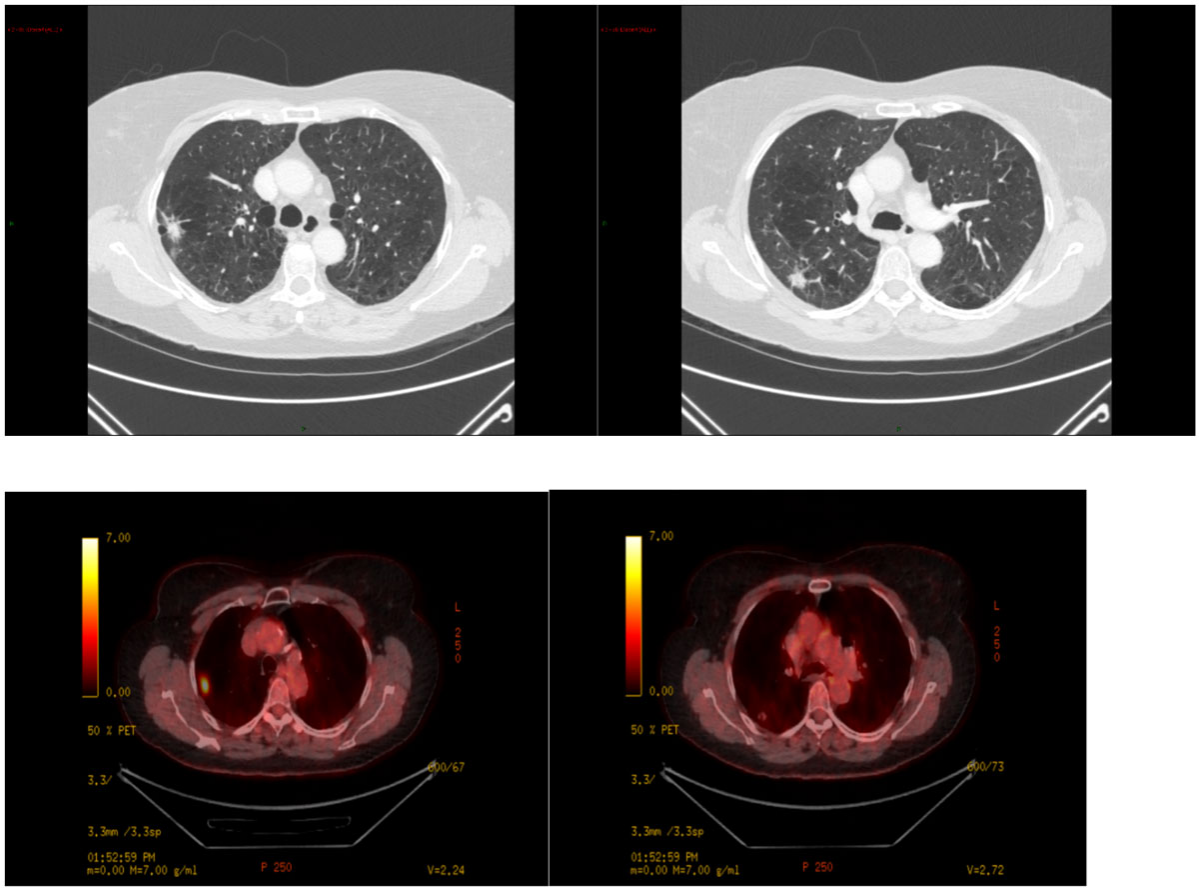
Two Right sided lung lesions. But only one is FDG Avid on the PET scan.
